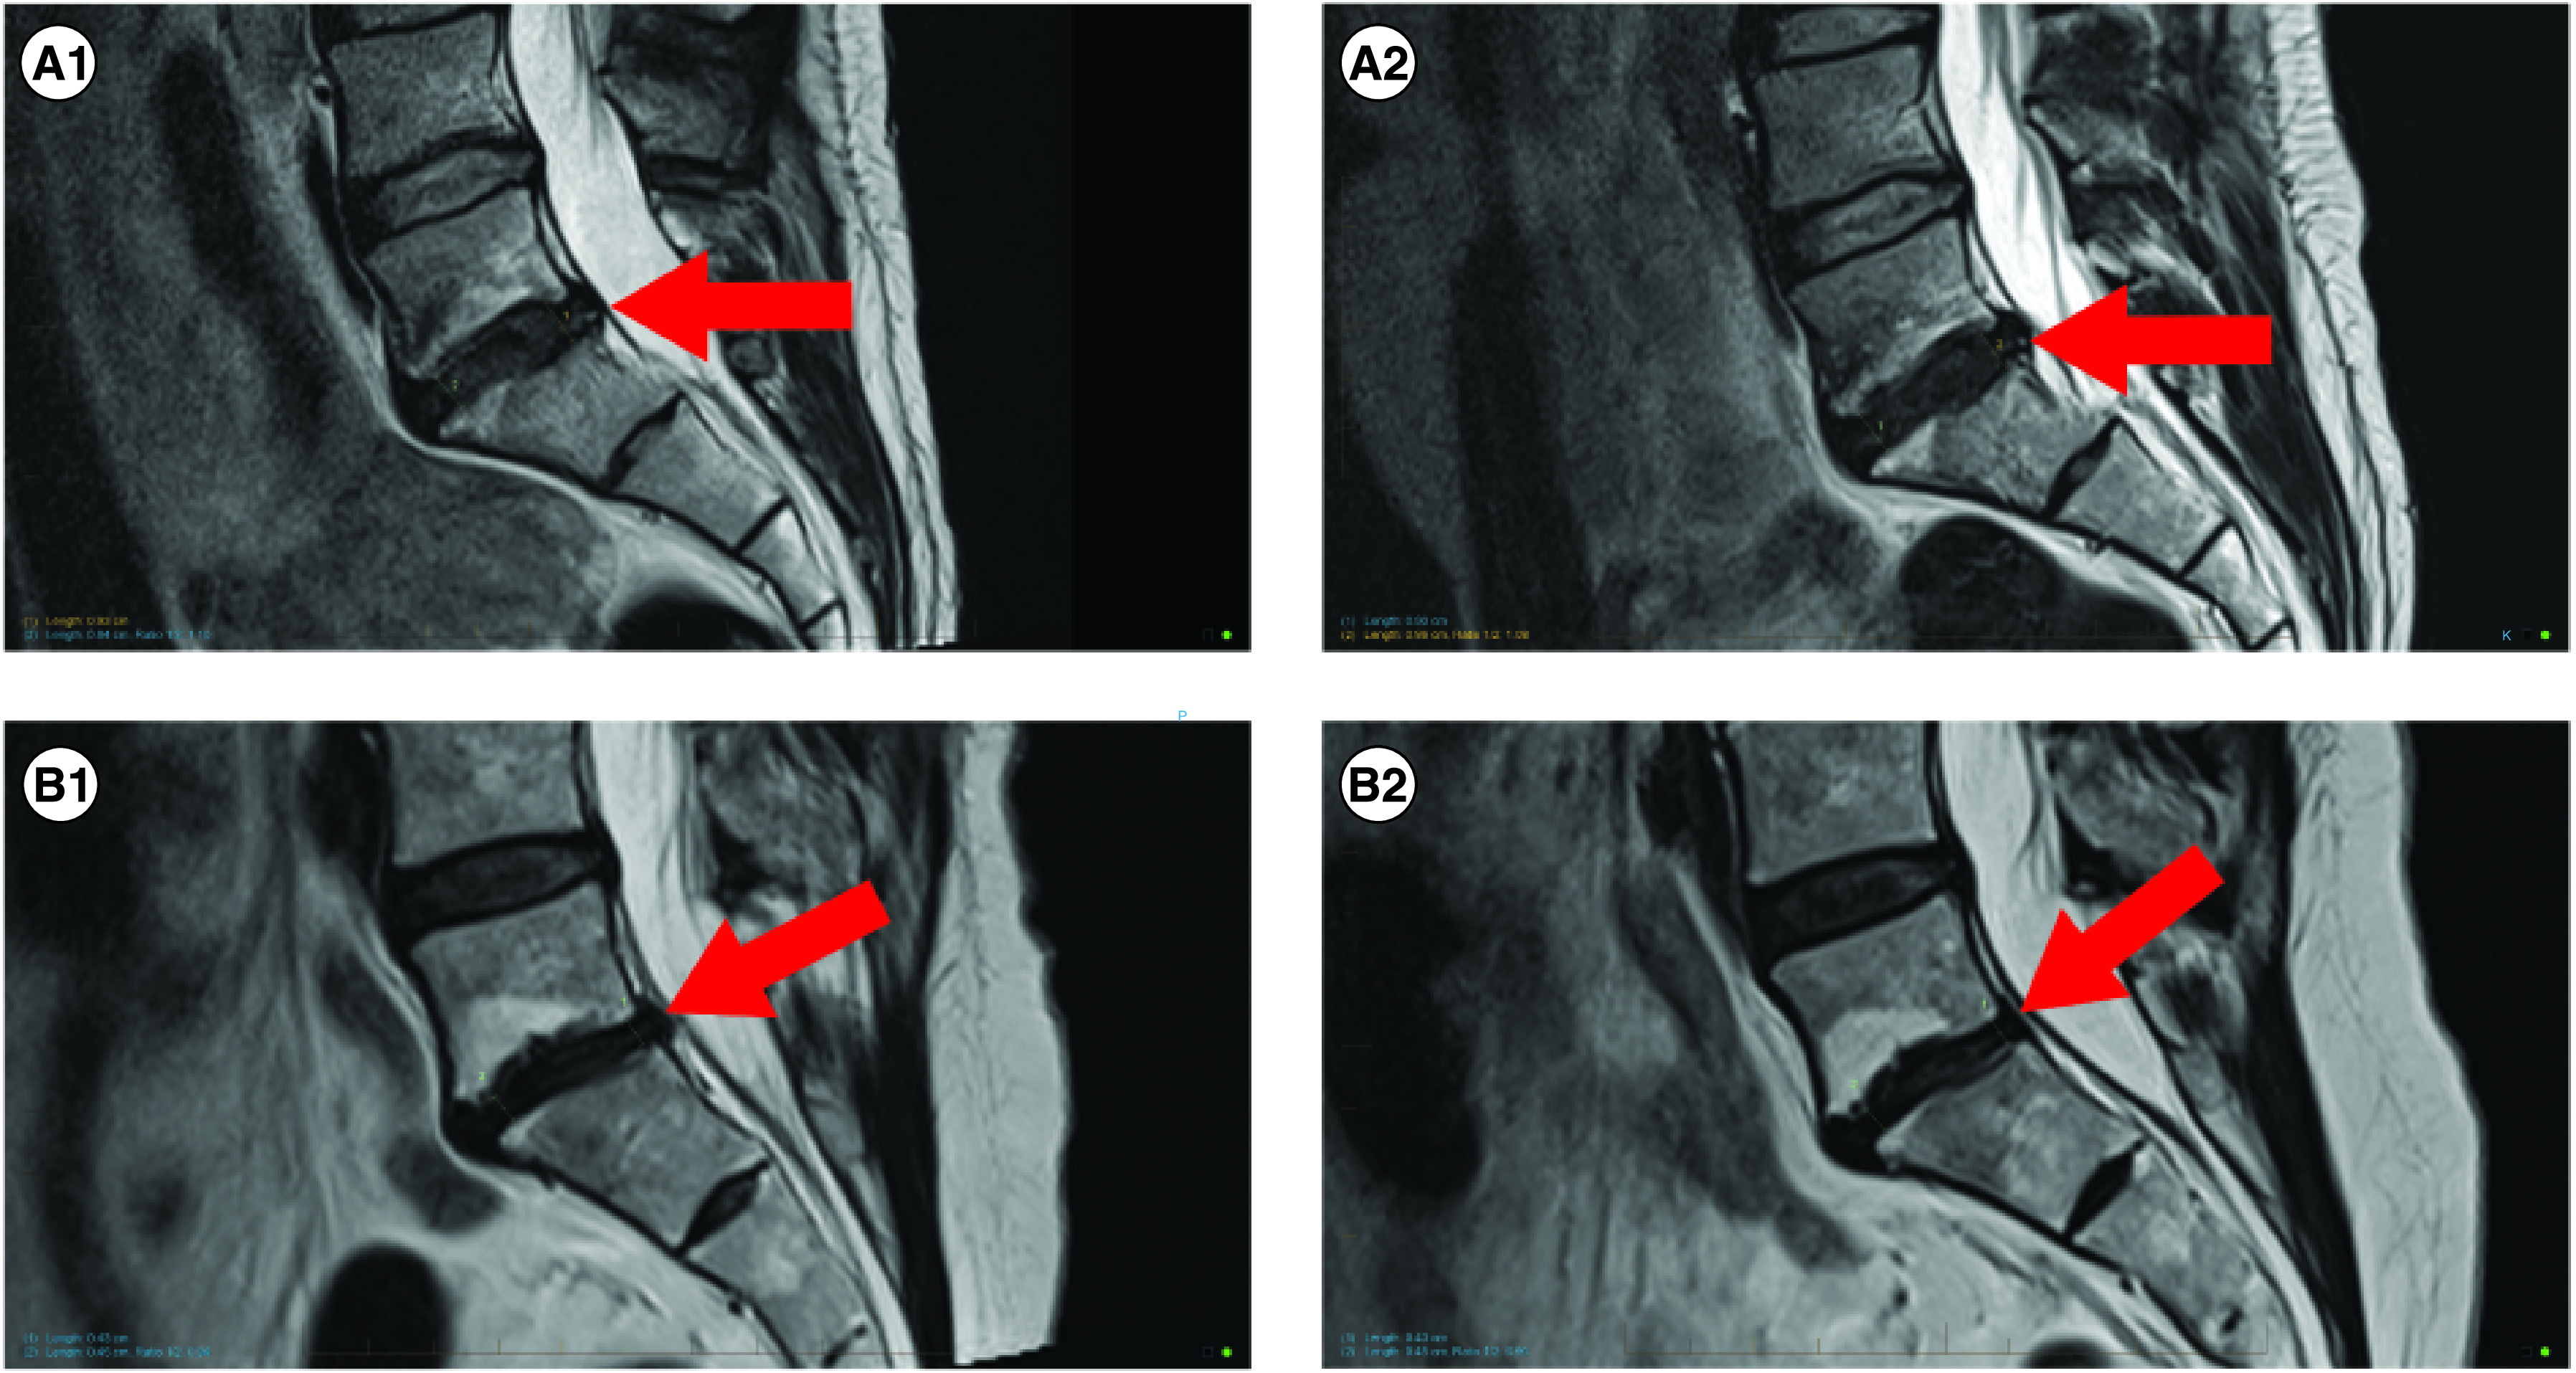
MRI images showing two participants at (i) baseline and (ii) 12 months post procedure. (A1 & 2) Represents participant 04 from Table 5 and shows no changes from baseline to follow-up. (A1) Participant 04, baseline. (A2) Participant 04, 12 months. (B1 & 2) Represents participant 07 from Table 5 and shows no changes in disc height, no progression of Modic 2 endplate changes and a reduction in disc protrusion size. (B1) Participant 05, baseline. (B2) Participant 05, 12 months.
